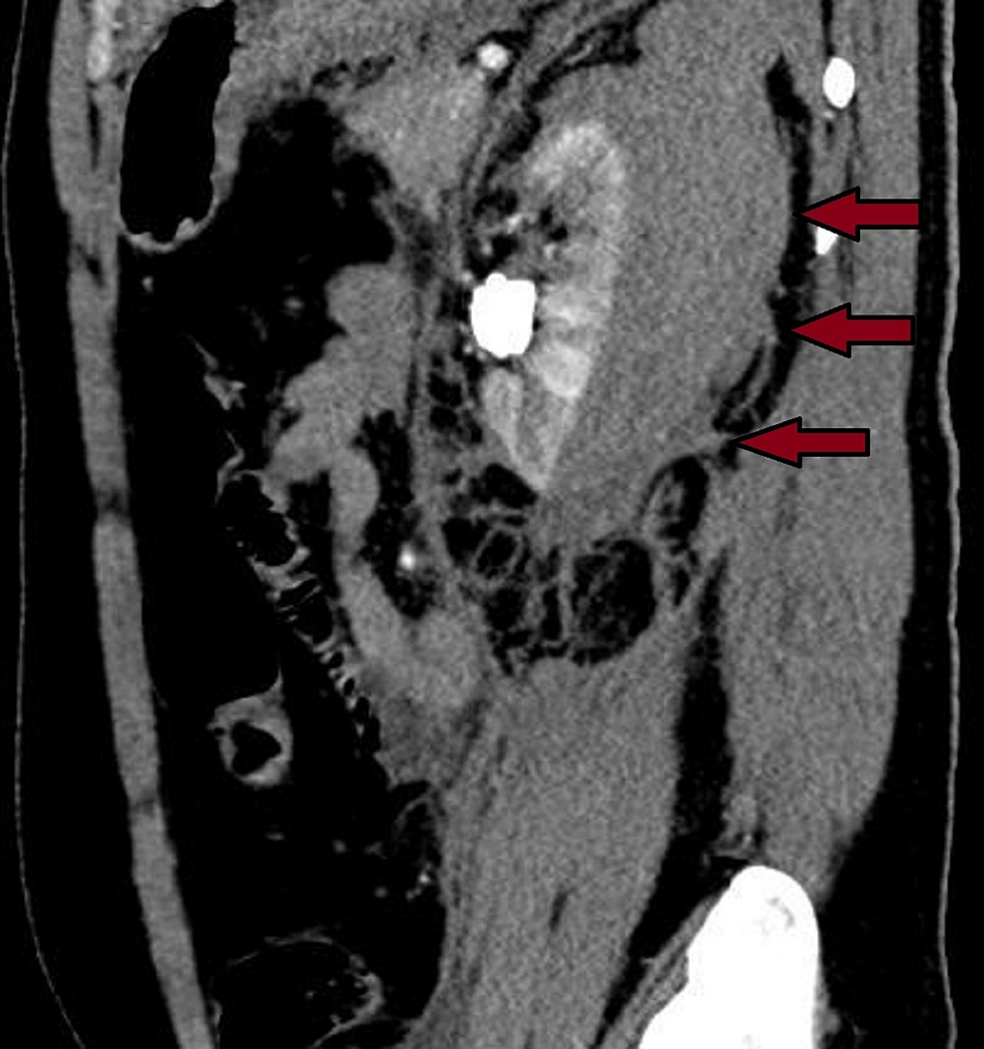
Computed tomography (CECT) of the abdomen showing massive left perinephric subcapsular hematoma (7.8 x 4.7 x 15.3 cm) without peritoneal collection.
